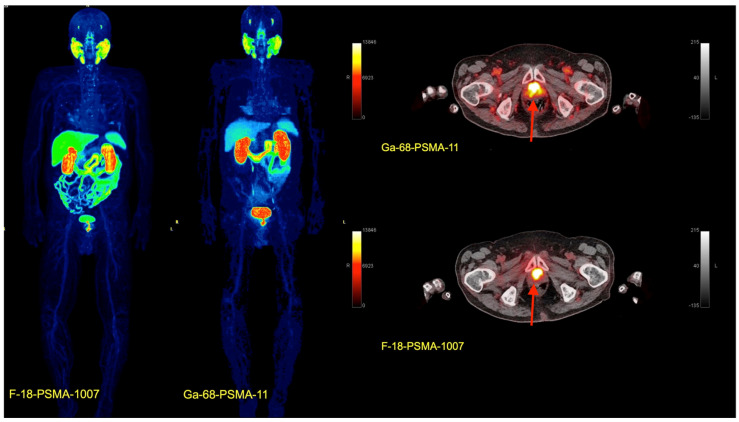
Example of a patient with biochemical recurrence of prostate cancer. MIP images 1.5 h after i.v. injection of [^18^F]PSMA-1007 (left side) and 1.5 h after application of [^68^Ga]Ga-PSMA-11 (right side) on the following day due to some non-specific bone uptake of [^18^F]PSMA-1007. Fused transversal images of a prostate cancer recurrence (red arrow) with both tracers. Of note is the different distribution of the two tracers. Enhanced hepatobiliary excretion of the [^18^F]PSMA-1007. Increased urinary excretion of [^68^Ga]Ga-PSMA-11.
